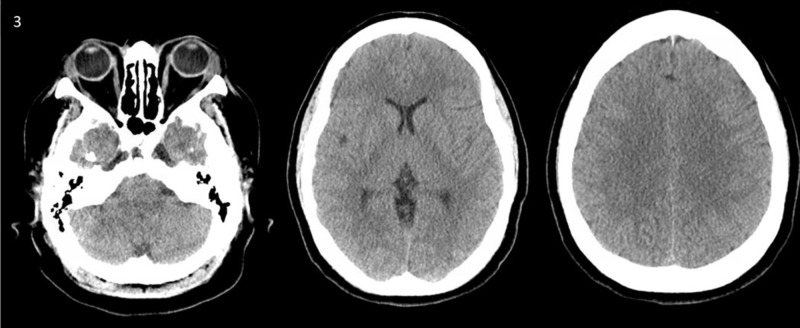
Picture demonstrated the resolution of the pneumocephalus (PNC). Follow up computed tomography scan after treatment showed resolved PNC.
